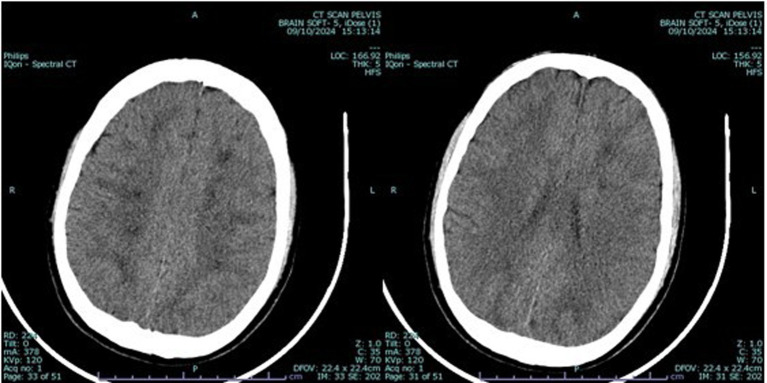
Different levels of axial brain computed tomography (CT) of Case 1 shows bilateral and symmetric multifocal hypodensities in the frontal and parietal subcortical (arrow) and deep white (arrow) predominantly in the centrum semiovale and involving the bilateral caudate heads, internal capsules, left thalamus, and cerebellum.
